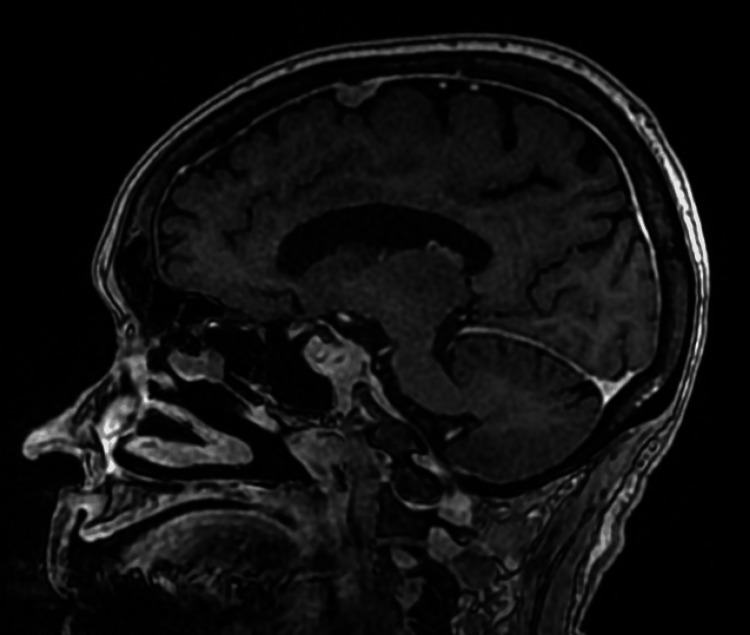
Post-contrast brain MRI showing diffuse smooth dural enhancement consistent with lymphomatous infiltration.
